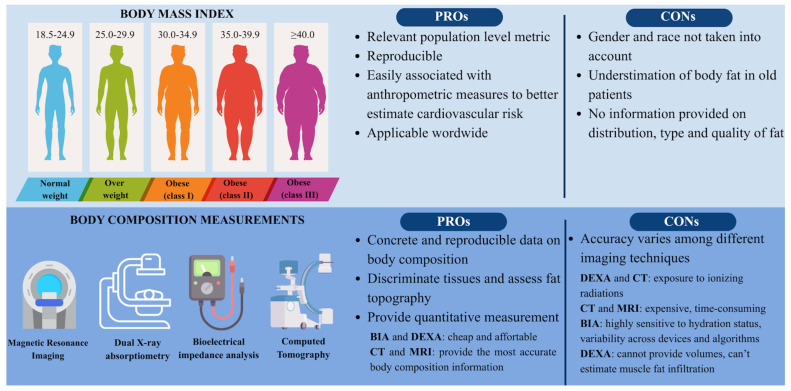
BMI is a simple and reproducible anthropometric measure that is widely accessible. However, BMI does not account for several important variables, including ethnicity, sex, and most critically, body composition, such as fat mass and lean mass distribution. Alternative modalities for assessing body composition include BIA, CT, DEXA, and MRI. These techniques offer objective and reproducible data on body composition. Nonetheless, each method has limitations. The accuracy of measurements may vary depending on the technique used; CT and DEXA involve exposure to ionizing radiation; DEXA does not provide information on tissue volumes or intramuscular fat infiltration and may yield less accurate results in individuals with severe obesity. Additionally, both CT and MRI are costly and not universally available, while BIA is influenced by the individual’s hydration status. BIA: bioelectrical impedance analysis, BMI: body mass index, CT: computed tomography, DEXA: dual-energy X-ray absorptiometry, MRI: magnetic resonance imaging.
